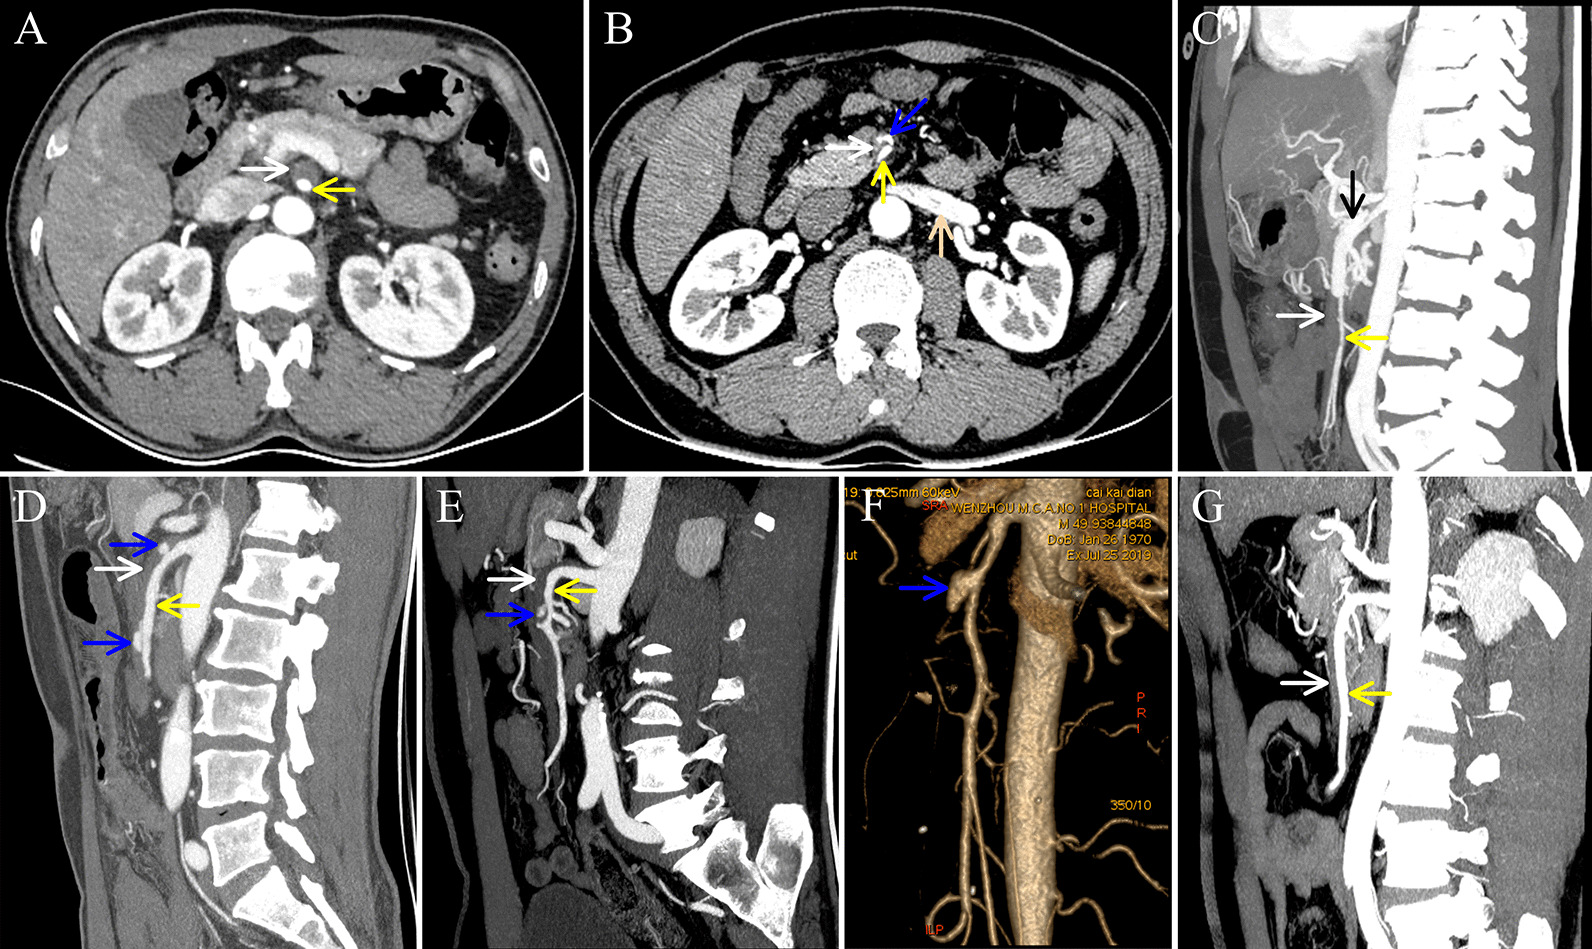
Features of Yun type IIb dissection. Axial CECT showing the true and thrombosed false lumens above the LRV plane (A) and the cyst-like residual false lumen in the thrombosed false lumen on the LRV (pink arrow) plane (B). Longitudinal CTA showing unthrombosed false lumen (black arrow) and thrombosed false lumen (C) and two cyst-like residual false lumens (D), one cyst-like residual false lumen (E), and no residual false lumen (G) in the thrombosed false lumen. CTA volume rendering (F) showing a cyst-like residual false lumen in the thrombosed false lumen. The yellow arrow represents the true lumen; the white arrow represents the thrombosed false lumen; and the blue arrow represents the cyst-like residual false lumen. *CECT* contrast-enhanced computed tomography, *LRV* left renal vein, *CTA* computed tomography angiography
